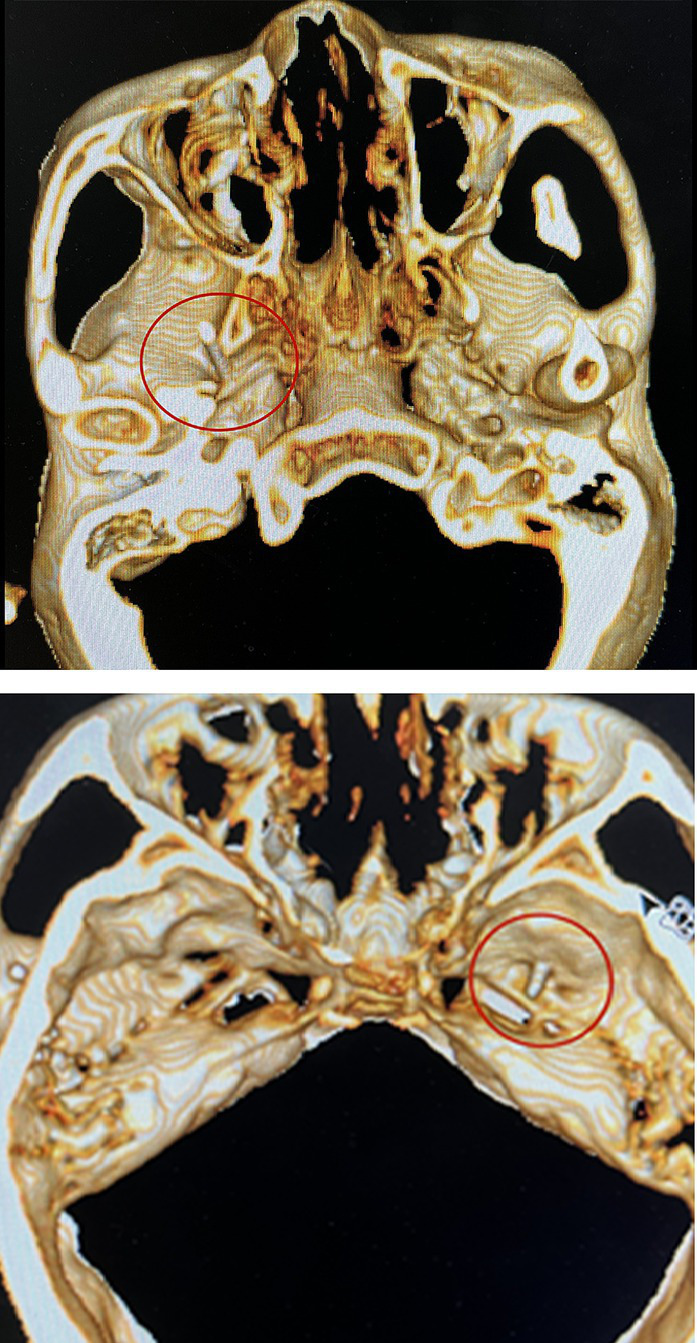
Radiofrequency trocar was used to enter the foramen ovale under the guidance of CT, and the radiofrequency machine was connected. Sensory stimulation at 50HZ and motor stimulation at 2HZ were given. After confirming the location, the patient was given three 90-s radiofrequency thermocoagulation at 80°C.
